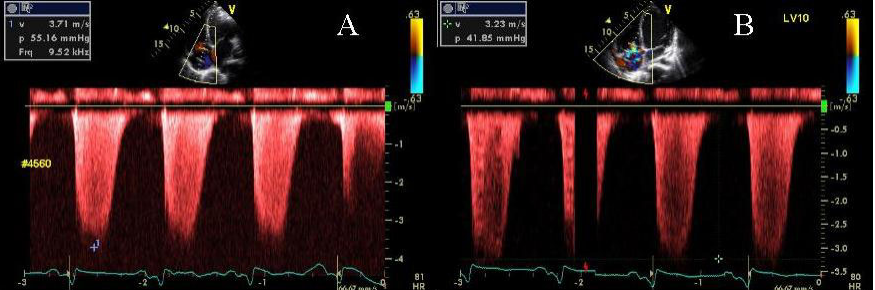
Continuous wave Doppler showing RV-RA gradient before (A) and after AV and VV optimization (B). Peak pulmonary artery systolic pressure reduced from 65 mm pre to 47 mm Hg post optimization.
